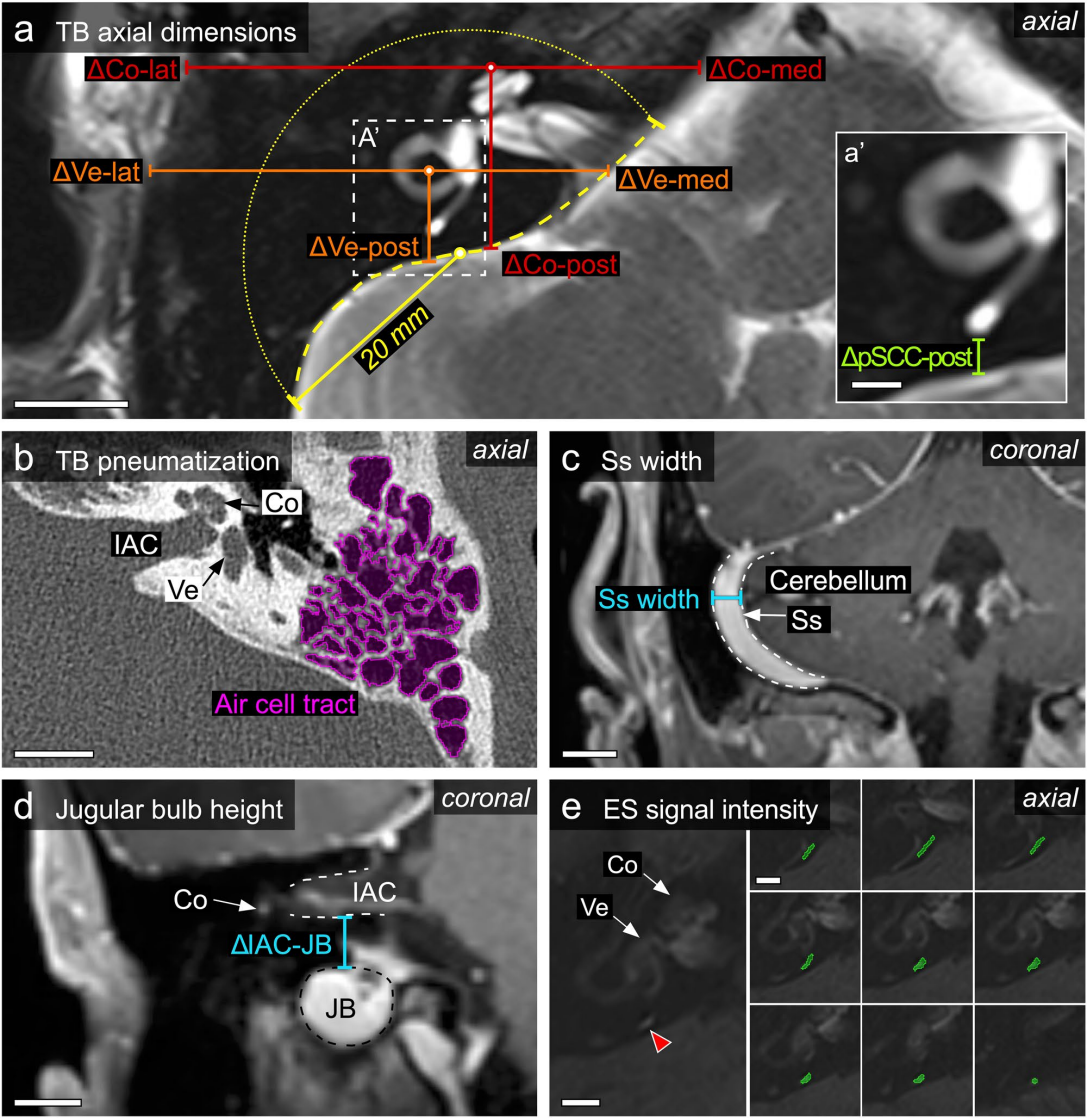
Summary of the TB parameters that were determined and the methods that were used. (a) Geometrical dimensions of TB (“bone thickness”) in the axial plane. ΔCo-lat/med/post: distance between the apex of the cochlea and the lateral, medial and posterior TB borders (red lines), respectively. ΔVe-lat/med/post: distance between the center of the horizontal semicircular canal and the lateral, medial and posterior TB borders (orange lines), respectively. To analyze the curvature of the posterior TB surface, a segment of the posterior TB border (yellow dashed line) within a circle (dotted yellow line) with a radius of 2 cm (yellow line) and its center at the exit of the vestibular aqueduct (yellow dot) was extracted. Scale bar 10 mm. (a′) ΔpSCC-post: distance between the most posterior edge of the posterior semicircular canal and the posterior TB surface. Scale bar 5 mm. (b) The pneumatized volume of the mastoid was determined by manually segmenting (outlined in magenta) and reconstructing its air cell tracts using HRCT imaging data. *Co* cochlea, *IAC* internal auditory canal, *Ve* vestibule. Scale bar 10 mm. (c) Sigmoid sinus (Ss; outlined by dashed white lines) width was measured at the level of the most lateral extension of the cerebellum. Scale bar 10 mm. (d) Height of the jugular bulb (JB) was determined by measuring its distance to the internal auditory canal. Co, cochlea. Scale bar 10 mm. (e) Inversion recovery SI of the ES (red arrowhead) was determined after manually segmenting the signal along its entire length (green outlines in serial image series). Scale bars 5 mm.
